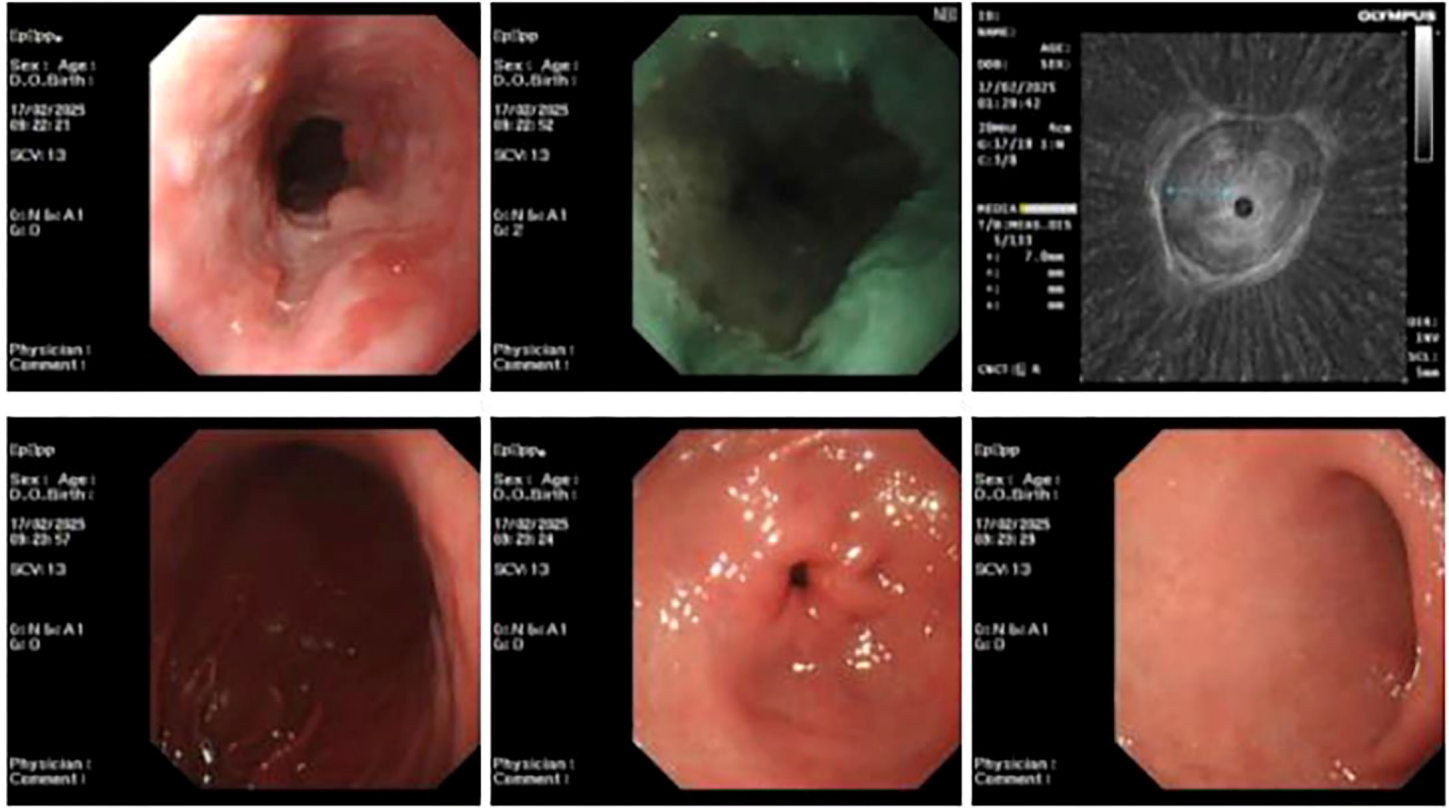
Ultrasound gastroscopy showing esophageal lesions at our hospital.
